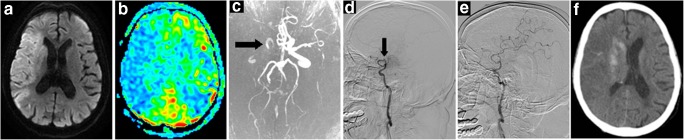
A 82-year-old woman (NO. 17 in Supplementary Tables 1 and 2) with a DWI-ASPECTS of 5 and an ASL-DWI mismatch ratio of 1.38 was treated by EVT alone and obtained successful reperfusion at 325 min from onset. This patient died of cerebral edema and herniation 10 days after intervention. a Infarction lesion presented on DWI (shown as high signal). b Hypoperfusion area shown on ASL-CBF map (blue). c Right ICA-MCA occlusion shown on MRA (black arrow). d DSA showing occlusion site of right ICA-MCA (black arrow) before EVT. e DSA showing reperfusion of occlusion vessel after EVT. f CT image at 24 h after EVT
